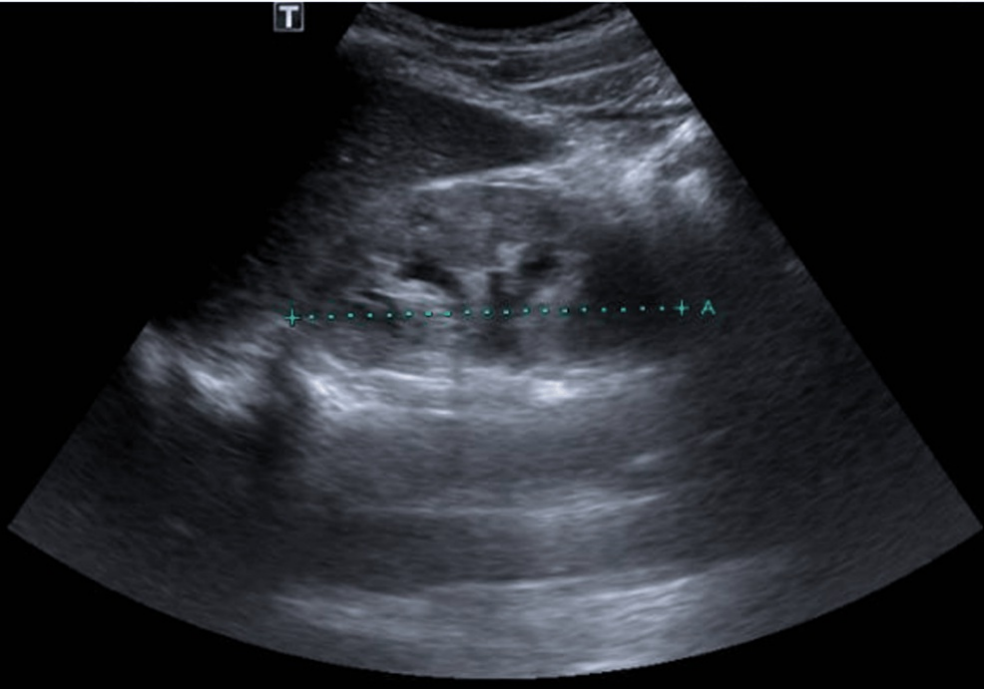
Renal ultrasound. Right kidney 9.6 cm, left kidney 9.1 cm long; no hydronephrosis, increase in echogenic pattern.
